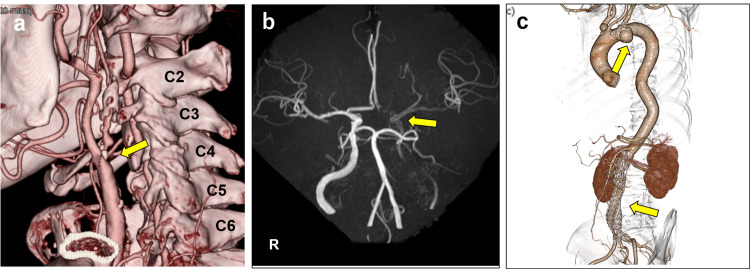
Asymptomatic severe stenosis of the left internal carotid artery (case one) a) Cervical contrast-enhanced 3D-CT angiography revealing severe stenosis of the left internal carotid artery (arrow). b) Head magnetic resonance angiography (MRA) showing poor visualization of the intracranial carotid artery and middle cerebral artery (arrow). c) Thoracoabdominal contrast-enhanced 3D-CT angiography demonstrating thoracic and abdominal aortic aneurysms post-stent graft placement (arrows)​.
